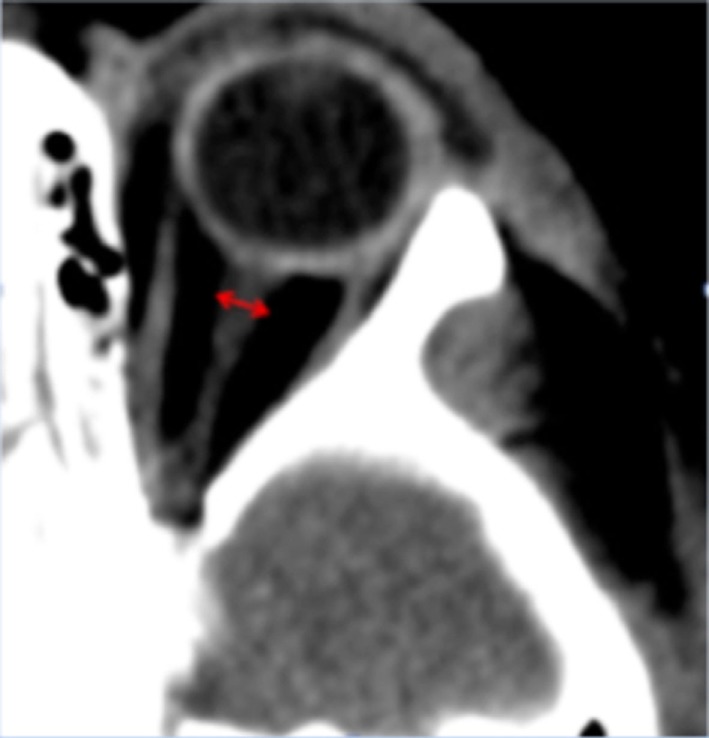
Representative computed tomography image of a pediatric patient showing measurement of the ONSD. The ONSD is typically measured 3 mm behind the insertion of the optic nerve into the globe, perpendicular to the long axis of the optic nerve.
